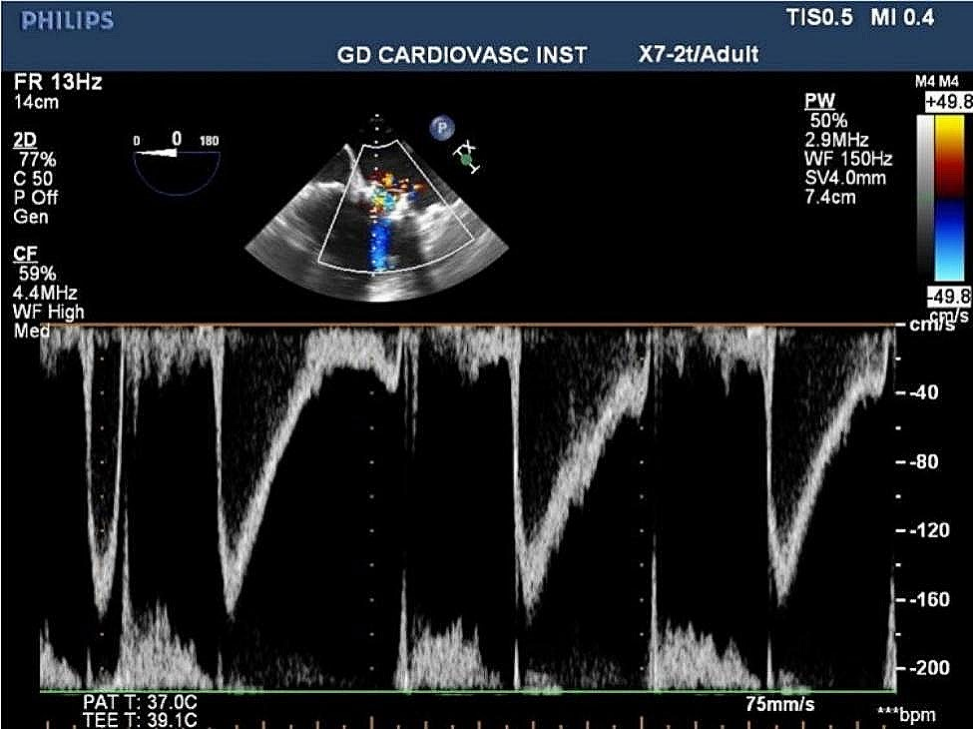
Postoperative transesophageal echocardiogram showed no significant mitral regurgitation
